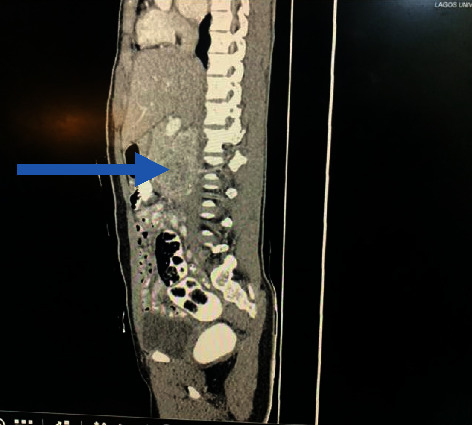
Contrast-enhanced sagittal reformatted computed tomography of the abdomen showing the posterior location of the mass (blue arrow).
